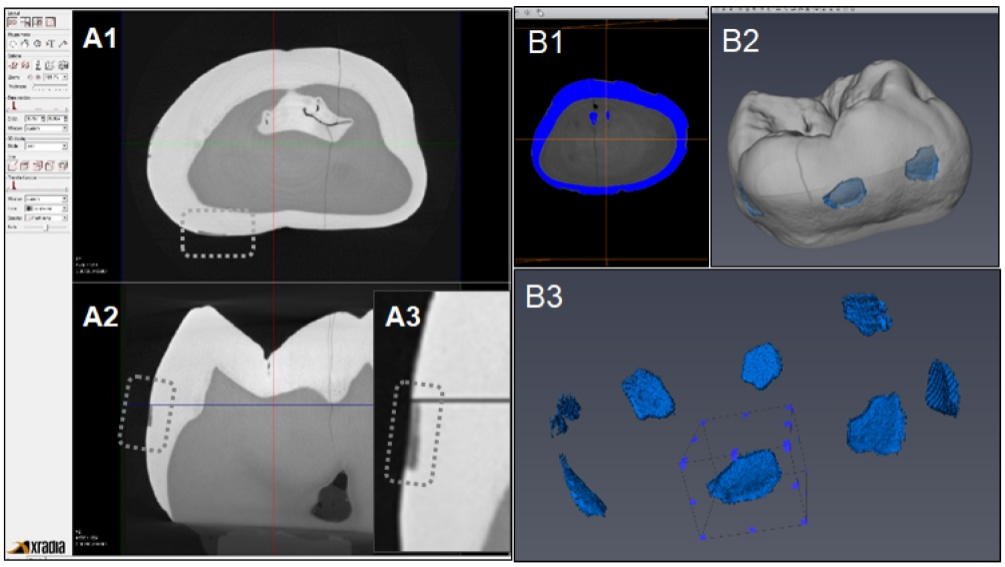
CT images acquisition and measurement of lesion volume using Aviso. A1: Xradia images reconstructed. A2: Shallow lesion were observed in the axial surfaces. A3: Enlarged image of lesion. B1: Original image for Aviso. B2: The 3D presentation of lesions. B3: The volume on each lesion were calculated.
